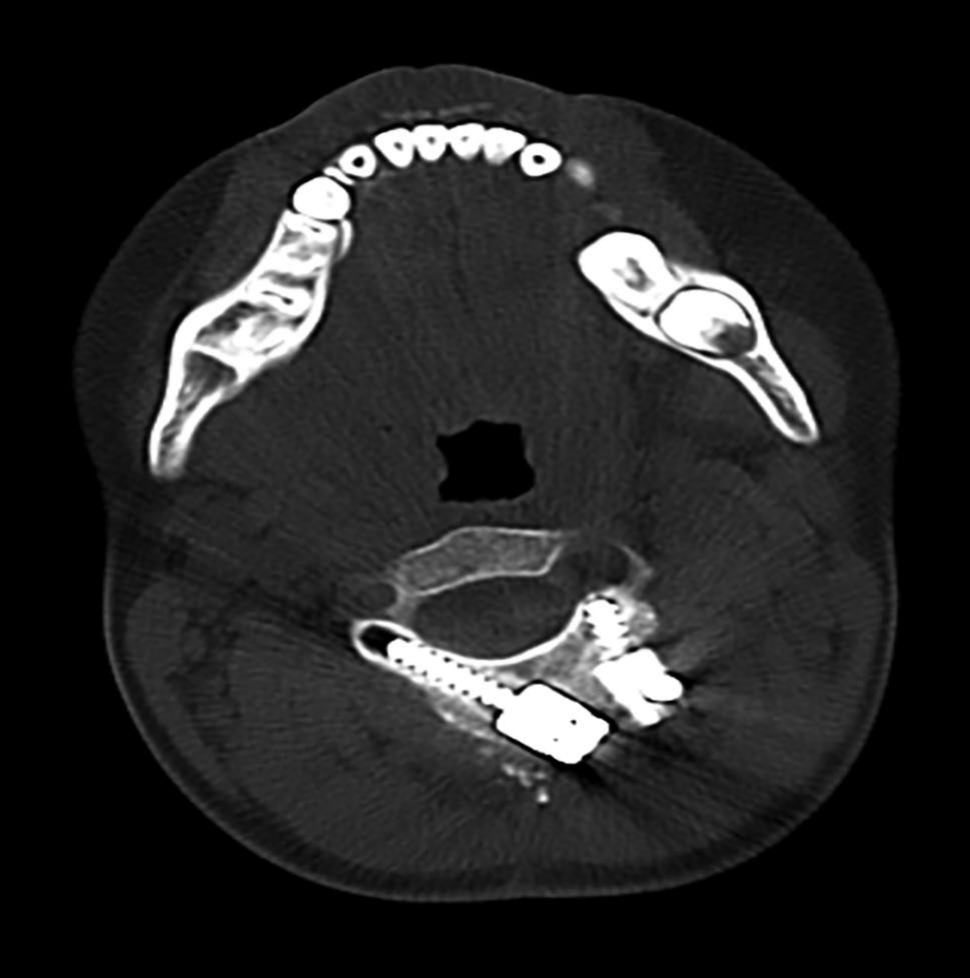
Translaminar C2 placement in patients with small C2 spinous process anatomy. An axial CT scan at the level of C2 is shown in a patient with a small C2 spinous process requiring placement of a notably shorter length left ipsilateral translaminar screw in a more ventral direction and lateral position, contralateral to a standard length right-sided translaminar screw in standard trajectory
